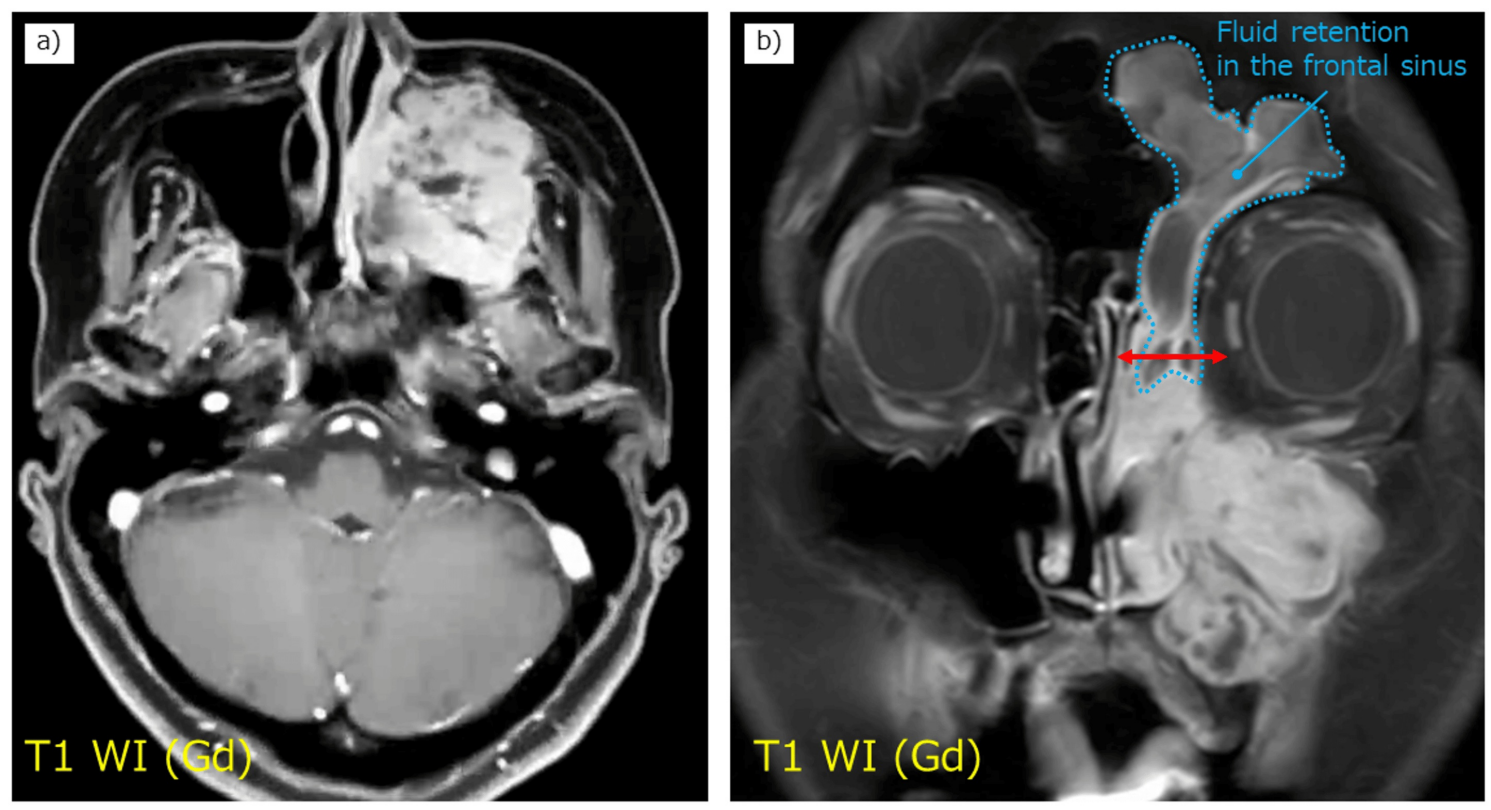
Preoperative contrast-enhanced magnetic resonance imaging scans. (a) Axial and (b) coronal T1-weighted images showing fluid accumulation (blue dotted line) in the frontal sinus without tumor infiltration. The images indicate that the osteotomy in the anterior, medial, and superior directions can be performed at the level of the ethmoid sinus, as indicated by the red line.
